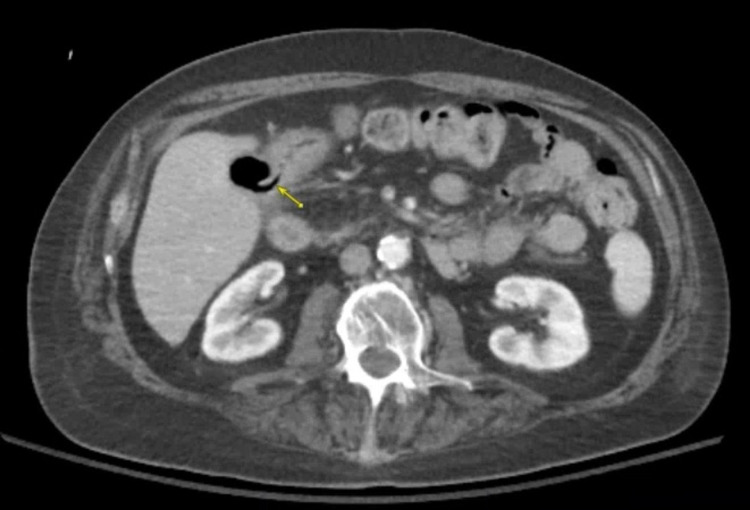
Computed tomography scan showing liner gas-filled tract (arrow) between the stomach and gall bladder, consistent with cholecystogastric fistula.
